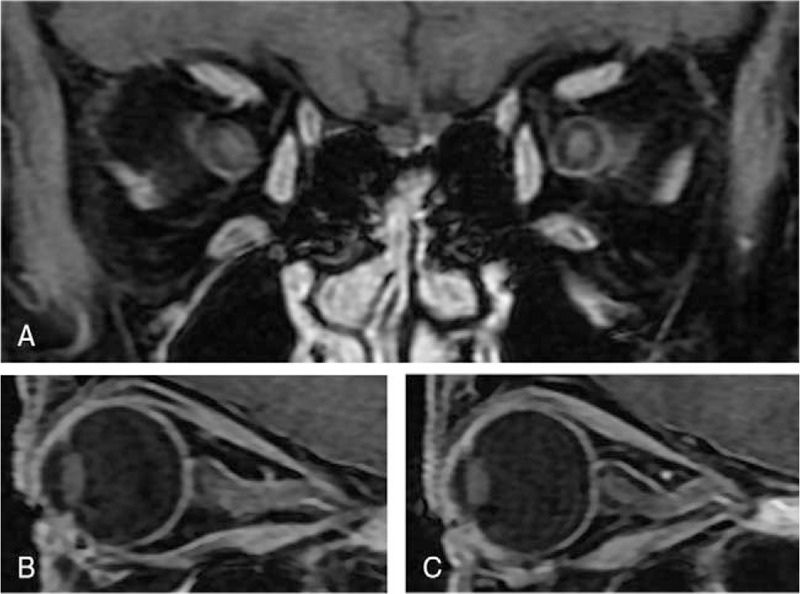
Postcontrast T1-weighted fat-suppressed magnetic resonance imaging (MRI) of the orbits. (A) Coronal MRI of the orbits reveals bilateral (but left dominant) uniform enhancement of the optic nerve. (B) Sagittal MRI of the right orbit reveals a slightly ill-defined appearance of the optic nerve and slight enhancement of optic nerve sheaths. (C) Sagittal MRI of the left orbit reveals uniform enhancement along with optic nerve sheaths.
